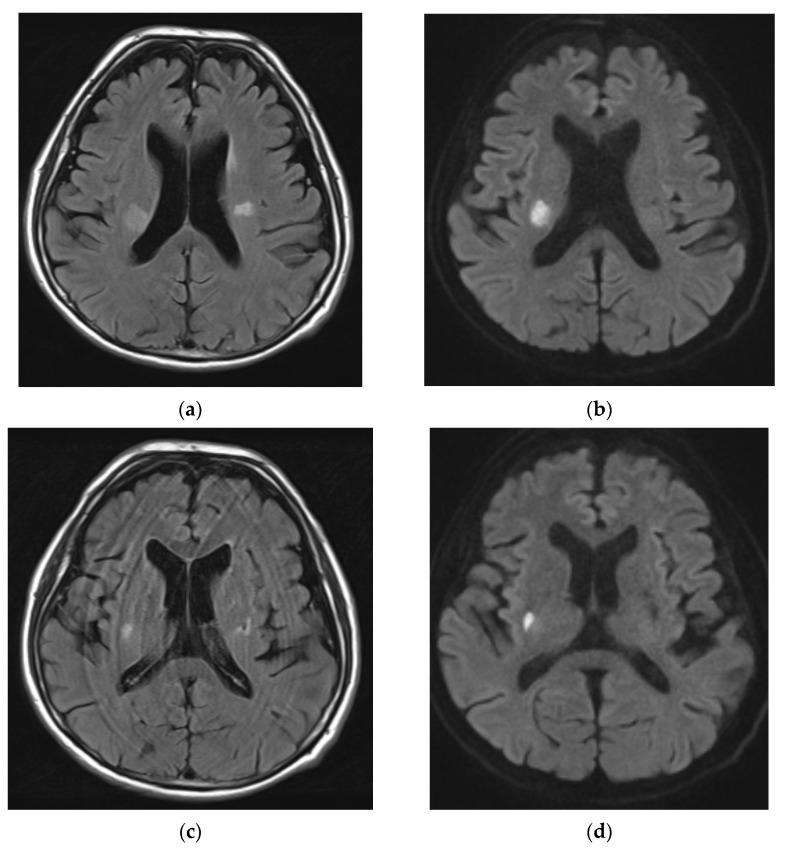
Brain MRI: axial flair (a,c) and DWI (b,d). This demonstrates the acute cerebral ischemia on the right AChA territory and an old ischemic stroke on the left AChA territory.
